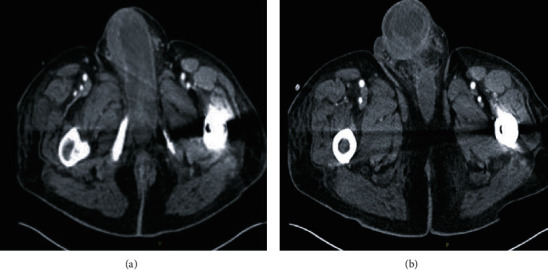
CT of the abdomen and pelvis with IV contrast obtained on postoperative day one demonstrating enlargement of the penis, likely consistent with metastatic, inflammatory, and postsurgical changes (a, b).
